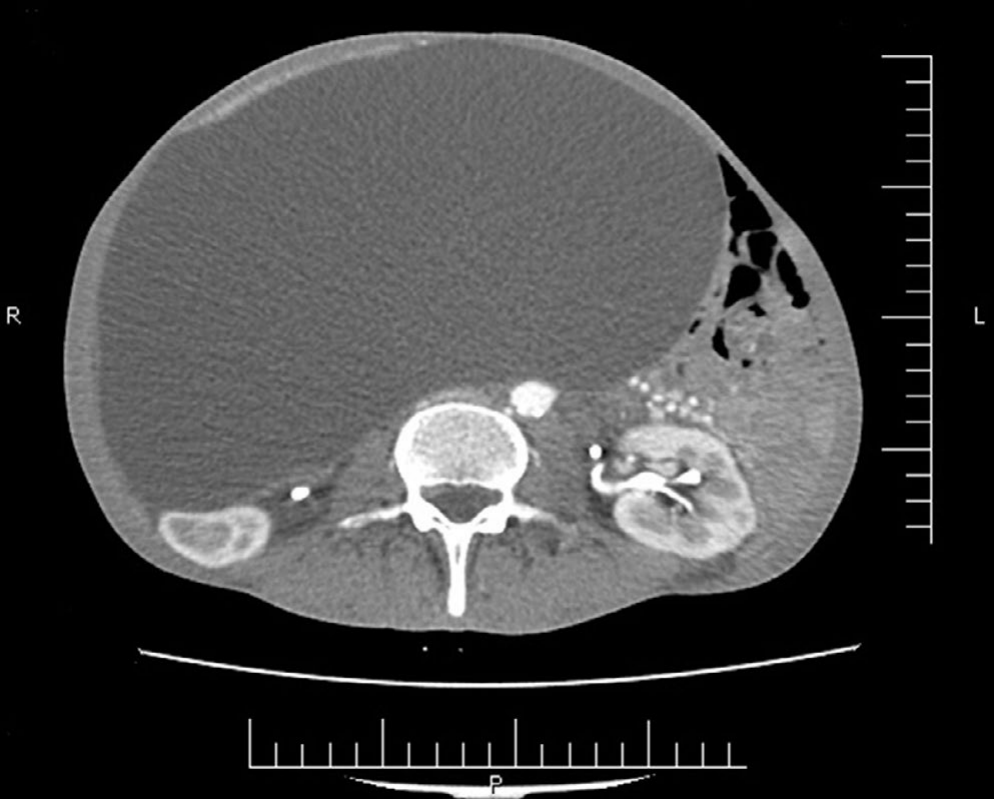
Axial abdominal computed tomography scan demonstrated a huge hypodense mass occupying the abdominal cavity and compressing intestinal loops
